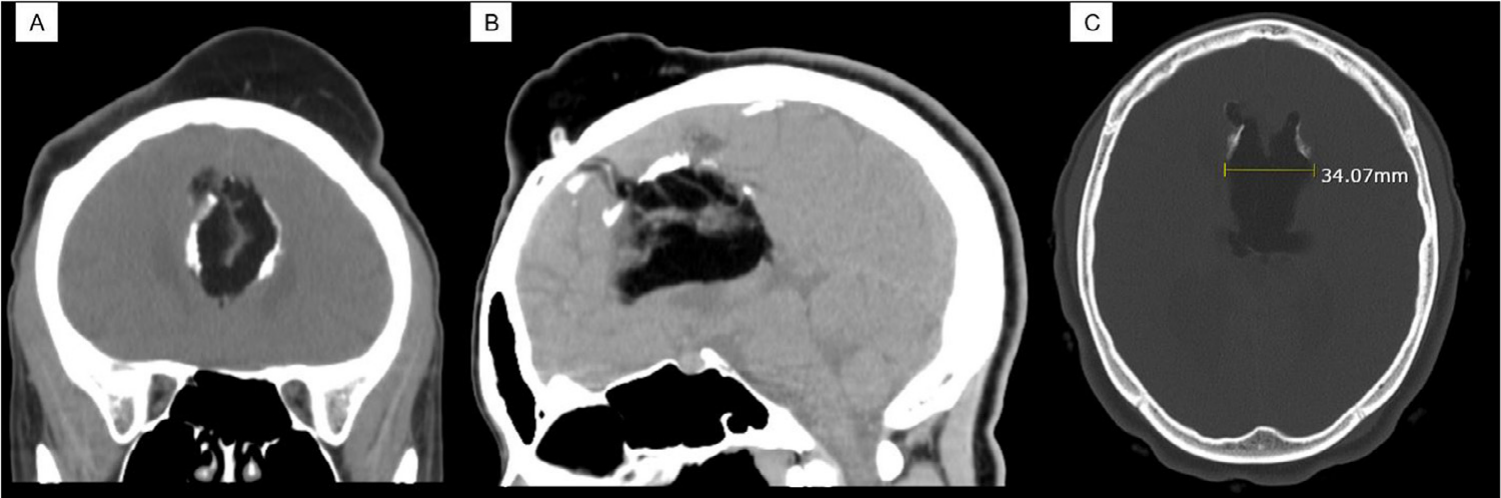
Head CT scan without contrast from November 2019. (A) Coronal view showing the interhemispheric lipoma. (B) Sagittal view showing the intracranial lipoma communicating with the scalp. (C) Axial view showing the interhemispheric and invasion into the body of the lateral ventricles bilaterally measuring about 3.4 cm.
